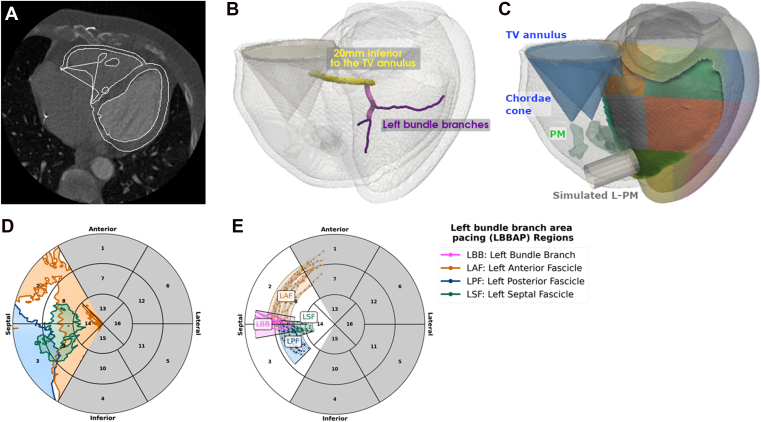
A: Cardiac computed tomography images were segmented and used to generate a (B) biventricular mesh, including the PMs, TV apparatus, and an estimation of the conduction system. C: American Heart Association regions were mapped onto the left ventricular epicardium and right ventricular (RV) septum. *Cylinders* representing the leadless pacemakers were virtually implanted across the RV septum surface. D: The >50% collision risk with the RV wall (*orange*), PM (*green*), and TV structures (*blue*). E: Left bundle branch area pacing regions were plotted. Non-RV septal regions are gray. L-PM = leadless pacemaker; PM = papillary muscles and moderator band; TV = tricuspid valve.
